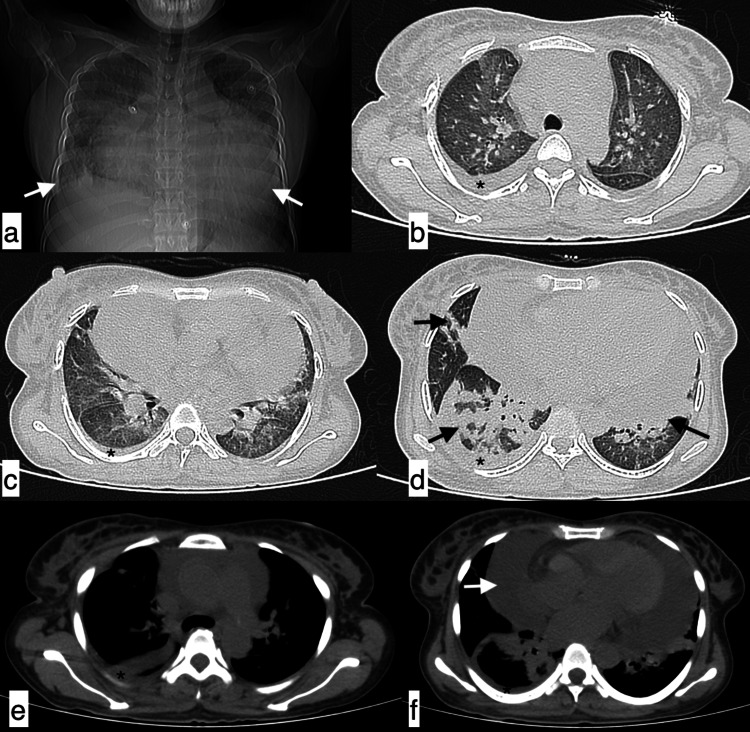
Pulmonary oedema with super-added infection A 25-year-old male patient with SLE presented with complaints of fever, shortness of breath, and cough of nine-day duration. The topogram (a) revealed gross pericardial effusion and blunting of bilateral costophrenic angles (white arrows) with diffuse ground-glass haziness in bilateral lung fields. Axial (b-f) HRCT chest images in the lung window (b-d) revealed diffuse ground-glass attenuation and interlobular septal thickening in bilateral lung fields, with patchy consolidation (black arrows) in the middle lobe, right lower lobe, and left lower lobe. Topogram findings were confirmed as mild right pleural effusion (b-f, asterisk) tracking along the fissure and cardiomegaly with massive pericardial effusion (f, white arrow). Features were suggestive of pulmonary oedema with super-added infection (Pseudomonas aeruginosa). SLE: systemic lupus erythematosus; HRCT: high-resolution CT
